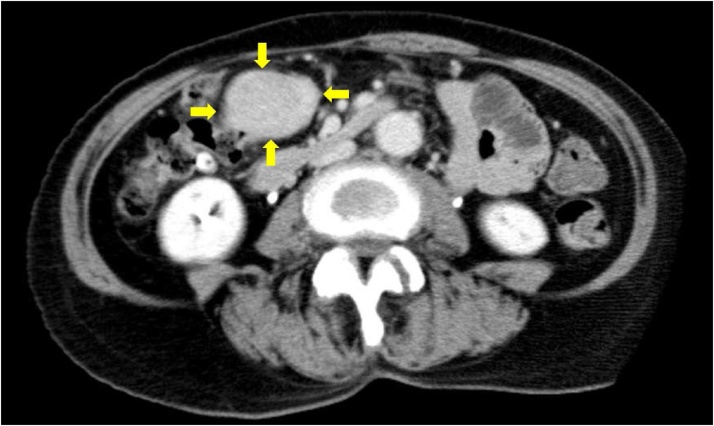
Abdominal contrast-enhanced computed tomography findings. A sharp border and homogeneous mass (arrows) are seen in the arterial phase, suggesting a gastrointestinal stromal tumor of the mesentery.
